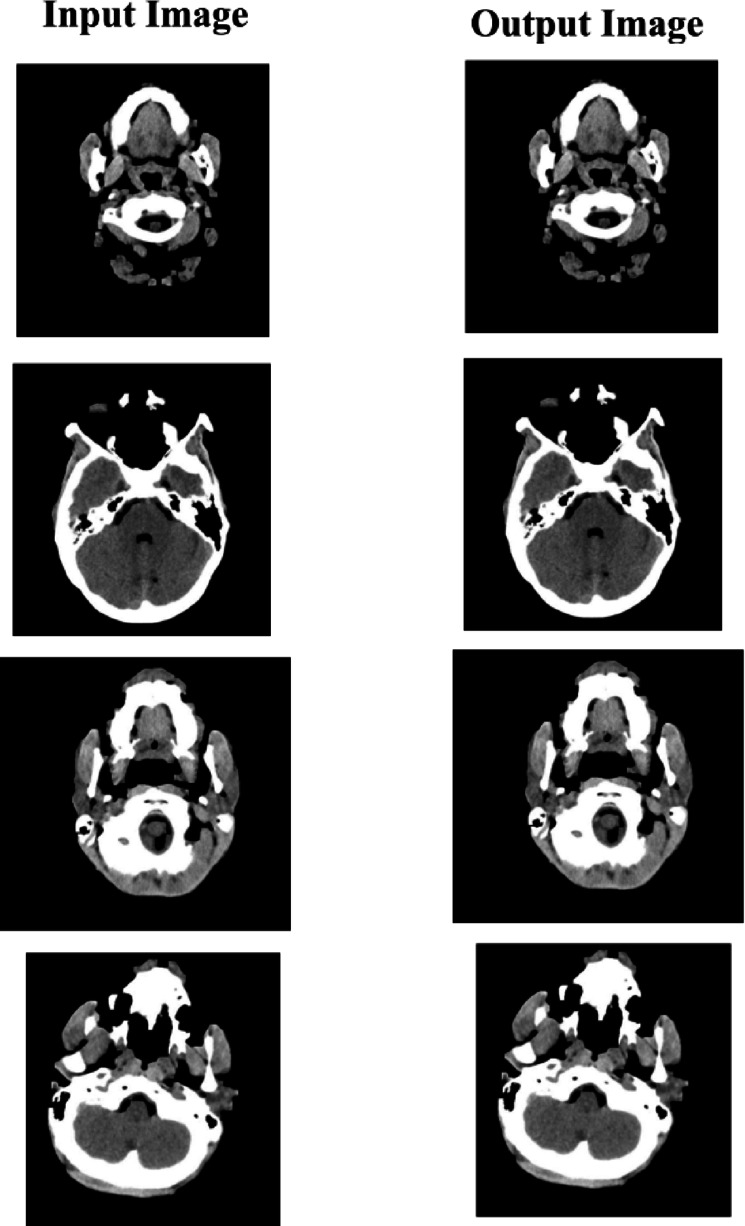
Anisotropic diffusion filtering applied to a CT brain scan image, effectively preserving the structural boundaries of brain tissues while reducing noise and enhancing tumor-relevant details.
